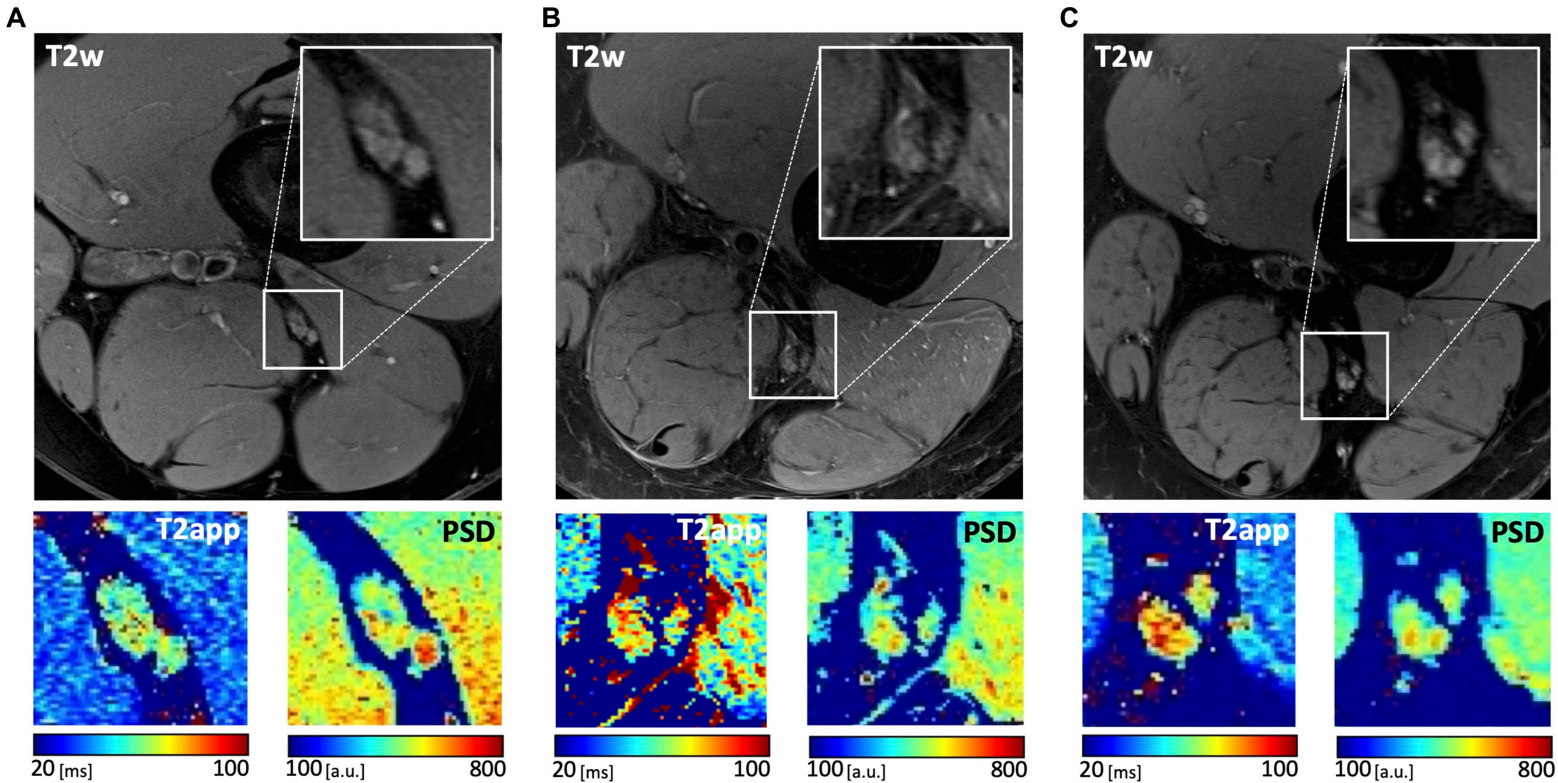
Representative images of the sciatic nerve at distal thigh level in a healthy participant (A) and in patient #14 at initial diagnosis (B) and at follow-up (C). Axial fat-saturated T2-weighted (T2w) images of the nerve as well as parameter maps of T2app and PSD with corresponding calibration bars are shown.
